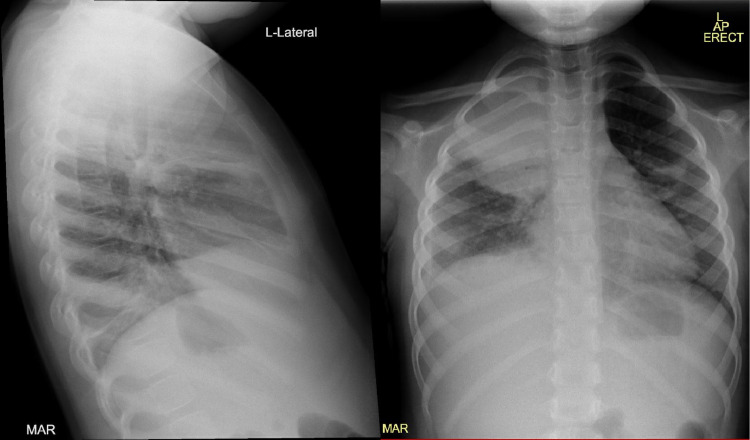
AP and lateral CXR showing right upper lobe consolidation and right pleural effusion. AP: anteroposterior; CXR: chest X-ray
